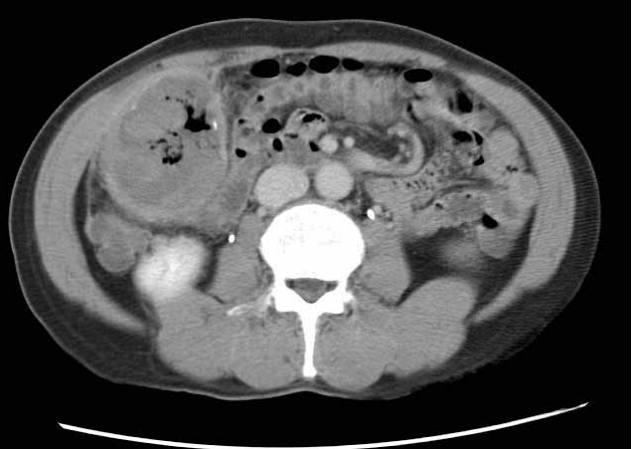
Enhanced abdominal CT scan of upper abdomen showing a 6 cm sized mass in the right lower quadrant of the abdomen at the first operation. The mass contained air bubbles, tiny calcifications and hematoma.
